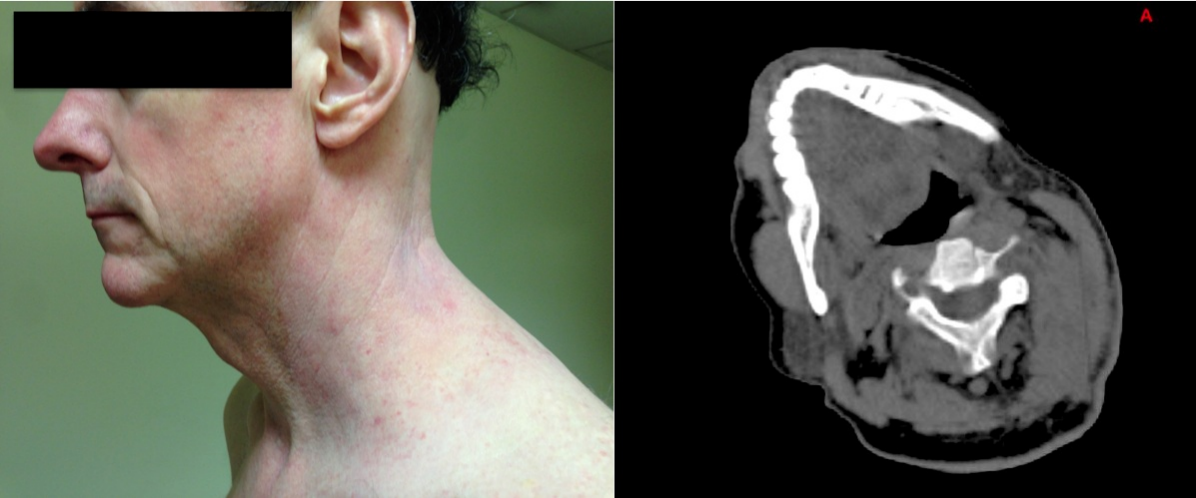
Tumor Response to Treatment The tumor is completely gone 5 months later clinically and on CT scan.
